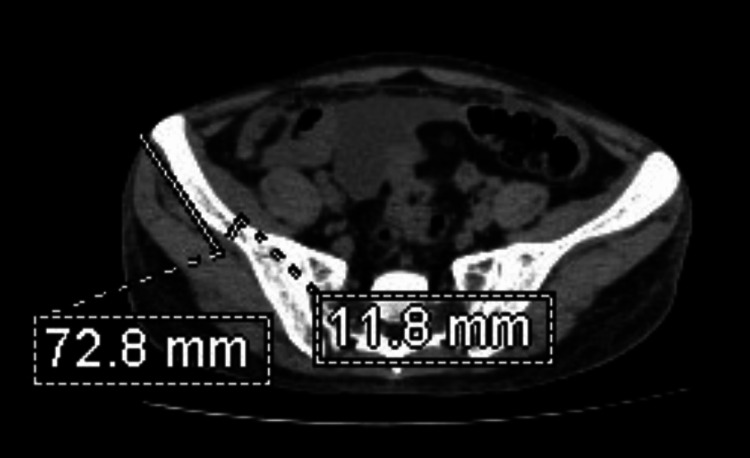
Axial CT section of the pelvis at the level of the sacral ala. Measurement of the iliac bone thickness at the midpoint of the line joining the anterior-superior iliac spine and the sacroiliac joint on the right side.
